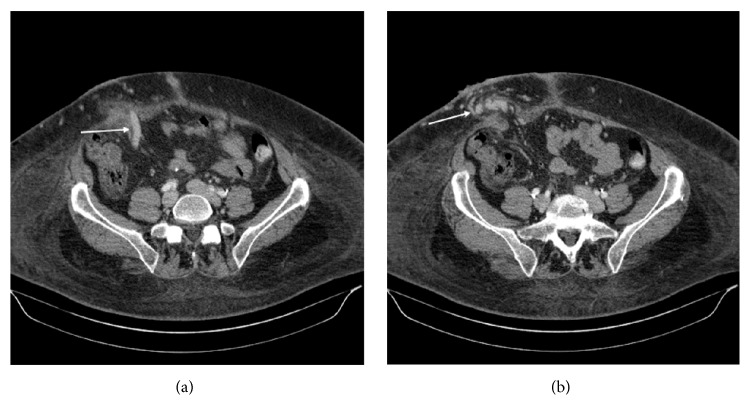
IV contrast-enhanced CT images of the abdomen and pelvic. (a) Large draining varix from the portal venous system (arrow). (b) Multiple varices within the right lower quadrant stoma (arrow).
